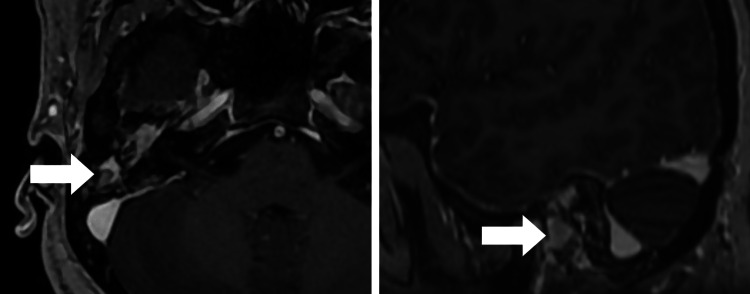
Axial and Sagittal T1-weighted post-contrast MR images showing heterogeneously enhancing components in the right middle ear cavity and mastoid air cells with a few non-enhancing and hyper-enhancing areas. MR: magnetic resonance.
